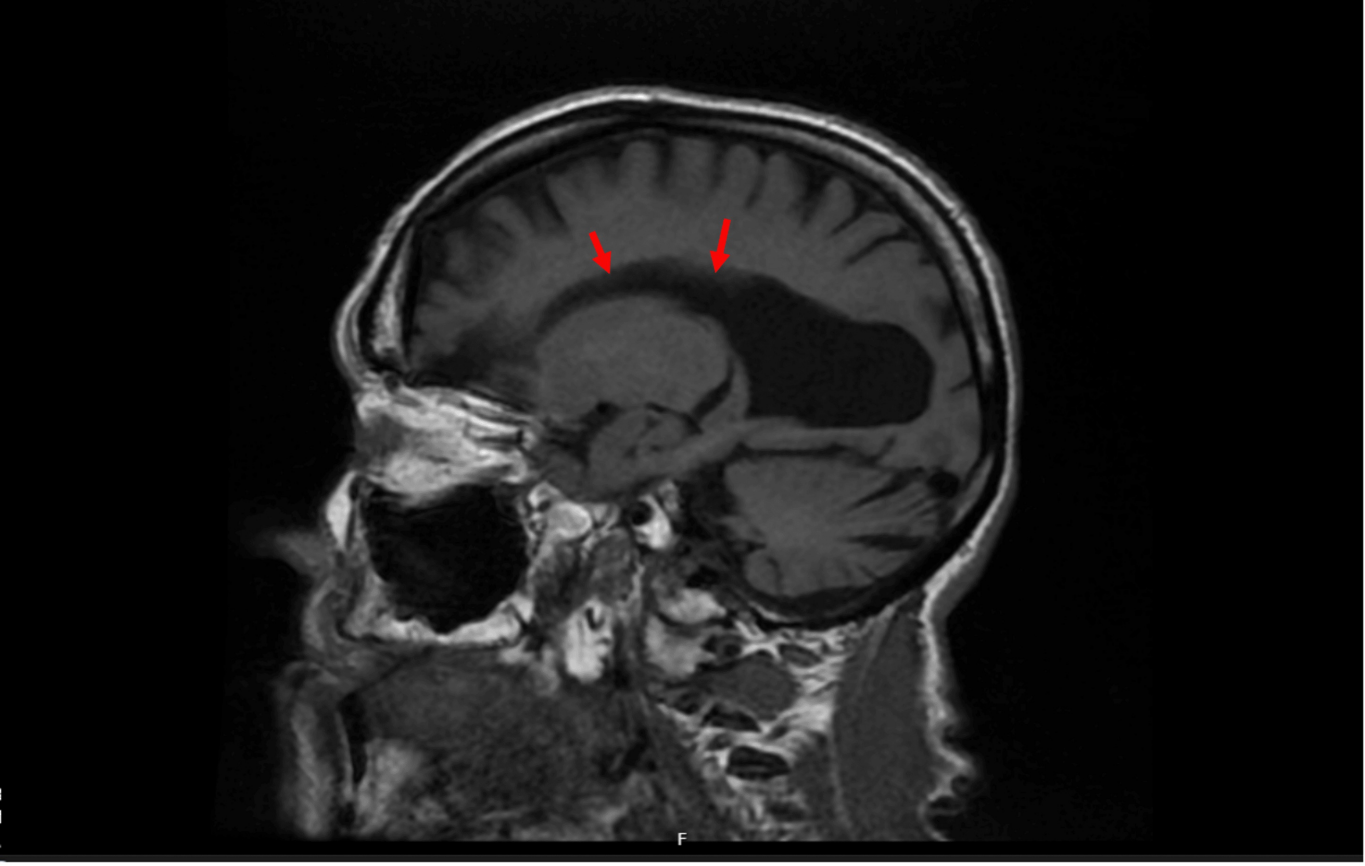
Sagittal view of MRI brain showing absence of the corpus callosum (red arrows) MRI - Magnetic Resonance Imaging
